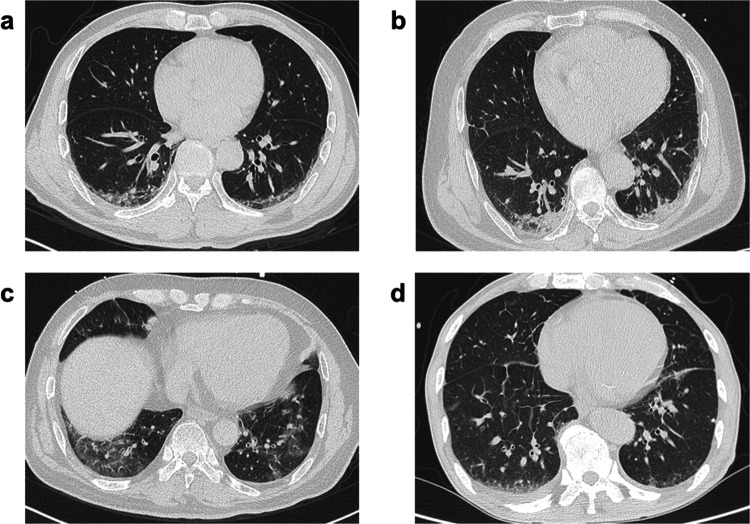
Representative chest CT images from the study cohort. (a) Subpleural ground-glass opacity. (b) Subpleural consolidation. (c) Diffuse ground-glass opacity with interlobular septal thickening. (d) Interlobular septal thickening.
